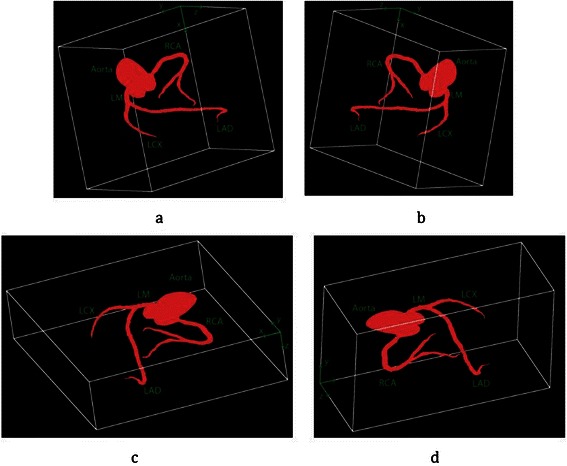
3D reconstruction of whole coronary arterial tree in CTA using the Intersection Tracking method. (a) - (d) Results of four different views.
